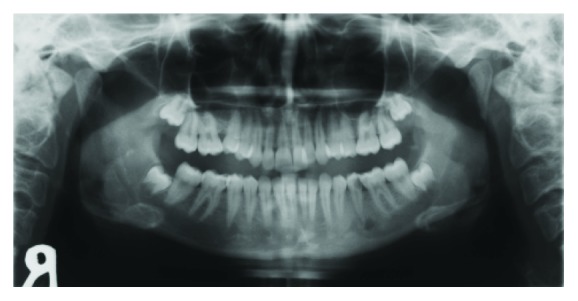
Orthopantomographic image showing a deep caries cavity in the left mandibular second premolar tooth and a radiolucent area in its apical region.
